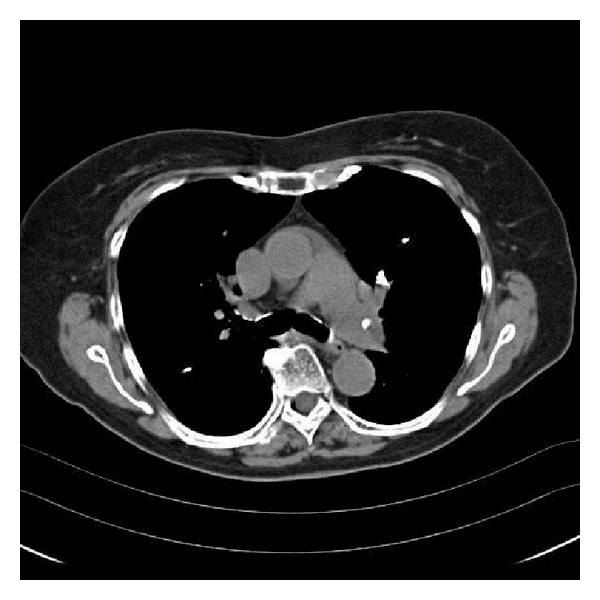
Computed tomography of chest without contrast depicting a clear left pulmonary artery filling defect.
